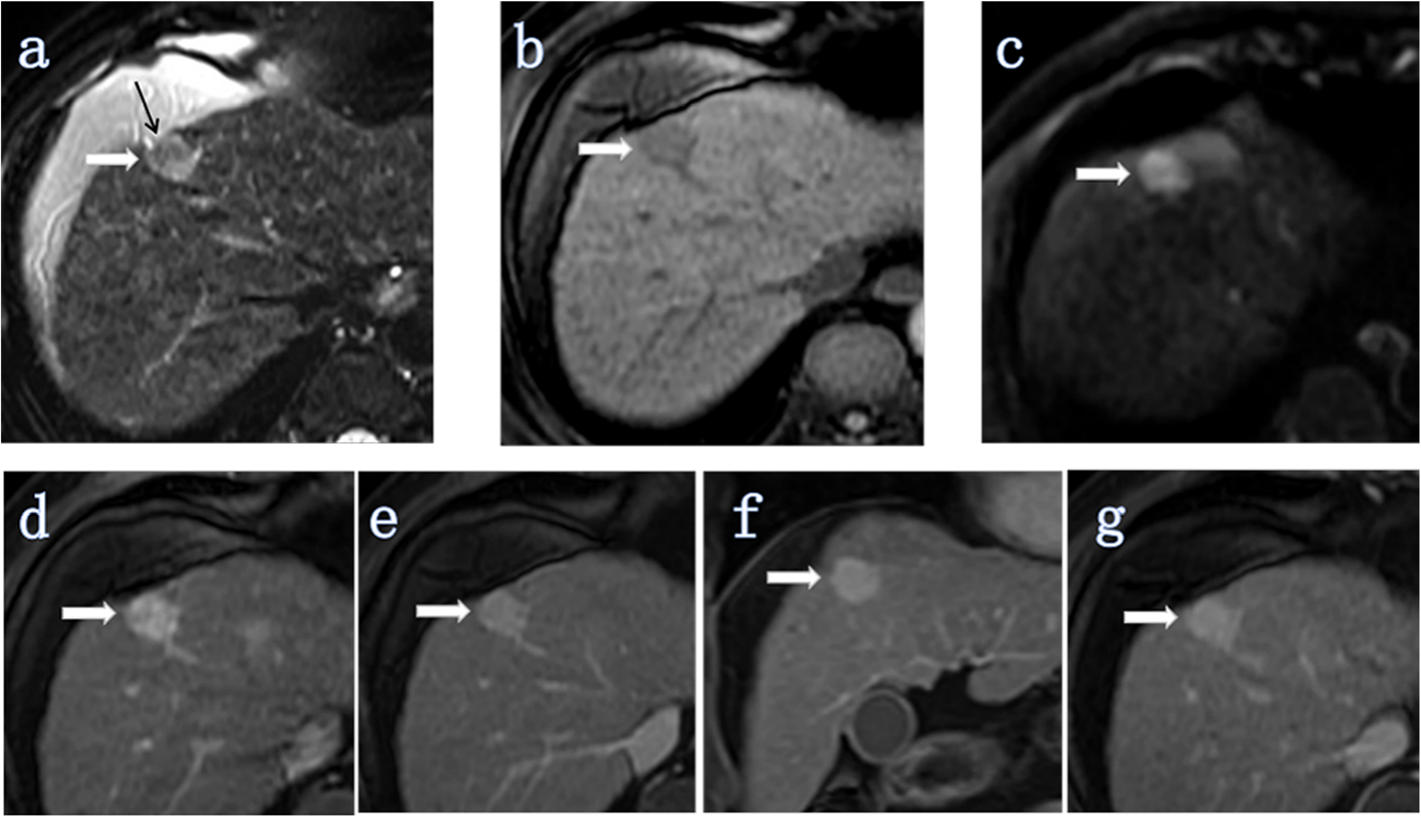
ICC (white thick arrow) without MVI in a 66-year-old man (tumor grade, G2; CA19–9, < 37 U/ml (20.6 U/ml)). Intrahepatic duct dilatation and visible hepatic artery penetration did not appear in all images of this lesion. a Axial T2-weighted image showing the signal of the tumor was unevenly high intensity and the retraction of the hepatic capsule adjacent to the tumor (black arrow). The tumor morphology was spherical. b Axial T1-weighted-FS image showing that the tumor was located in segment IV of the liver. c Axial DW image (b = 500 s/mm^2^) showing a uniform high signal. d-g Images during the arterial to delayed phase showing that the tumor showed continuous overall enhancement (high signal). The maximum diameter of the tumor was 1.89 cm and the maximum enhancement edge thickness was 1.89 cm, and the arterial edge enhancement ratio was 100% (1.89/1.89)
